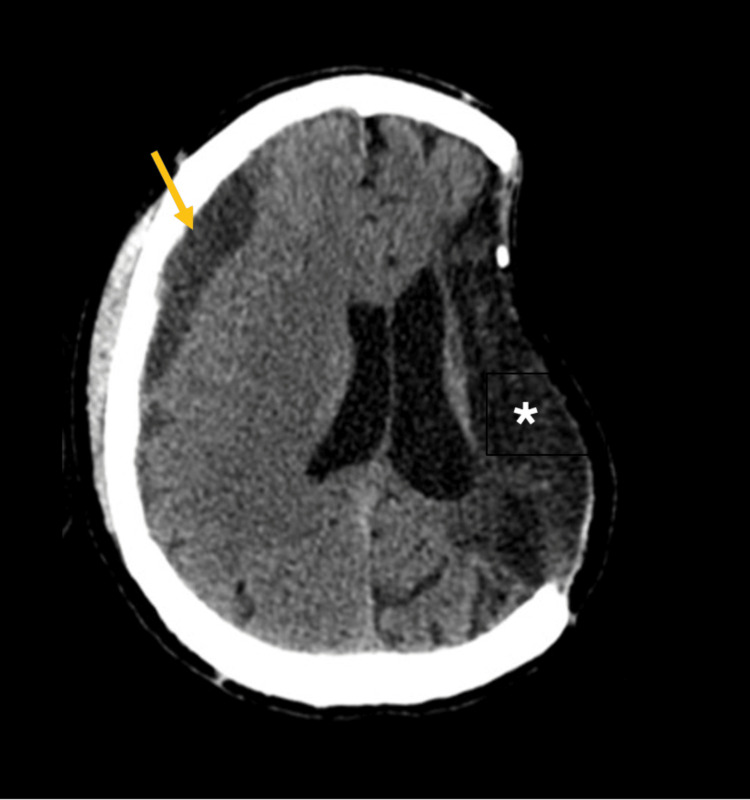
Non-contrast cranial computed tomography Axial non-contrast head CT demonstrates postoperative changes related to prior left-sided decompressive craniectomy (asterisk) with a right frontoparietal chronic subdural hematoma (arrow).
